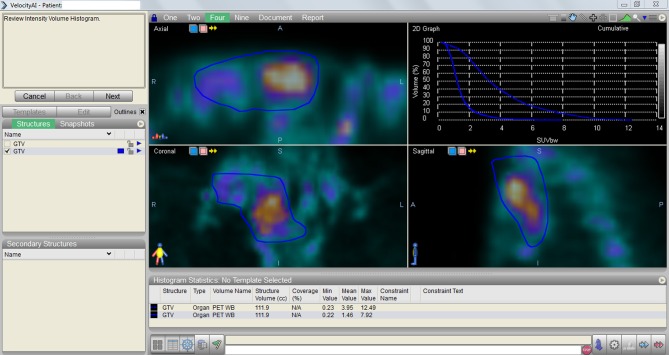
Displayed the assessment graphic containing the data obtained by the comparison of pre-treatment vs. post-treatment PET/CT findings by using Velocity AI “Navigation” tool.
